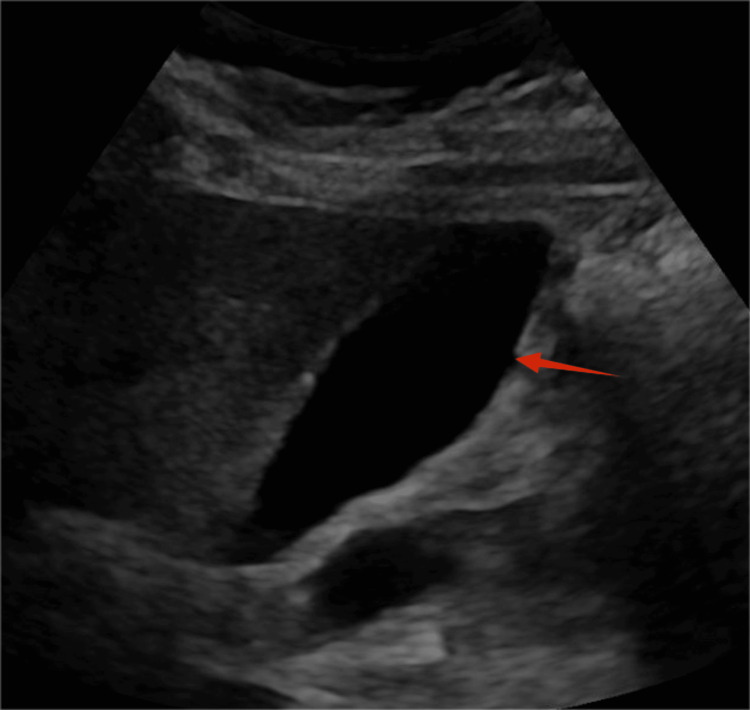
Right upper quadrant ultrasound revealing a normal-appearing gallbladder or absence of gallstones or wall thickening
